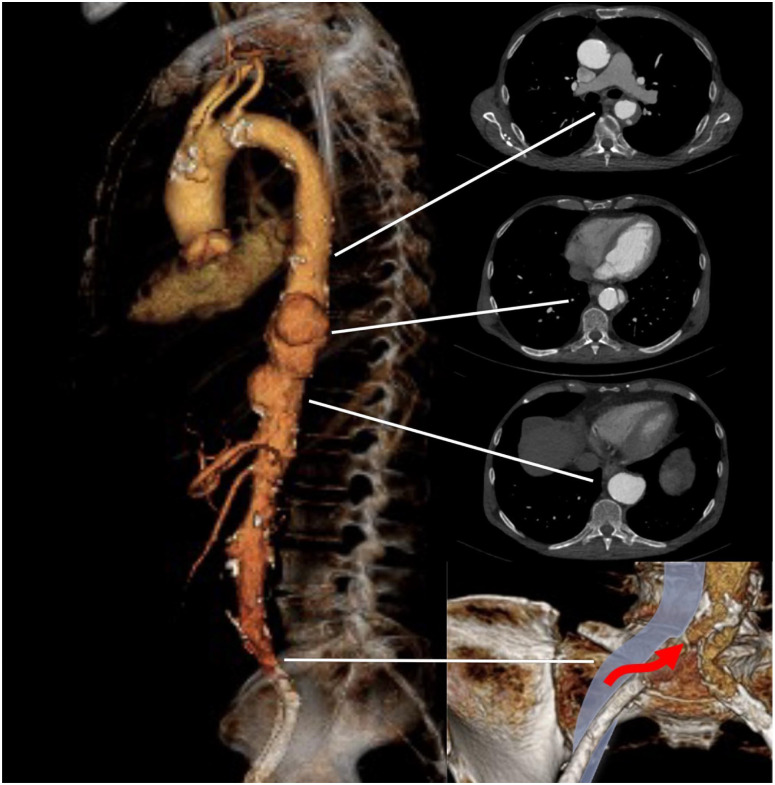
Three-dimensional rendering of the preoperative computed tomography scan with 3 axial views. Red arrow indicates the access point to the common iliac artery from the common iliac vein.
